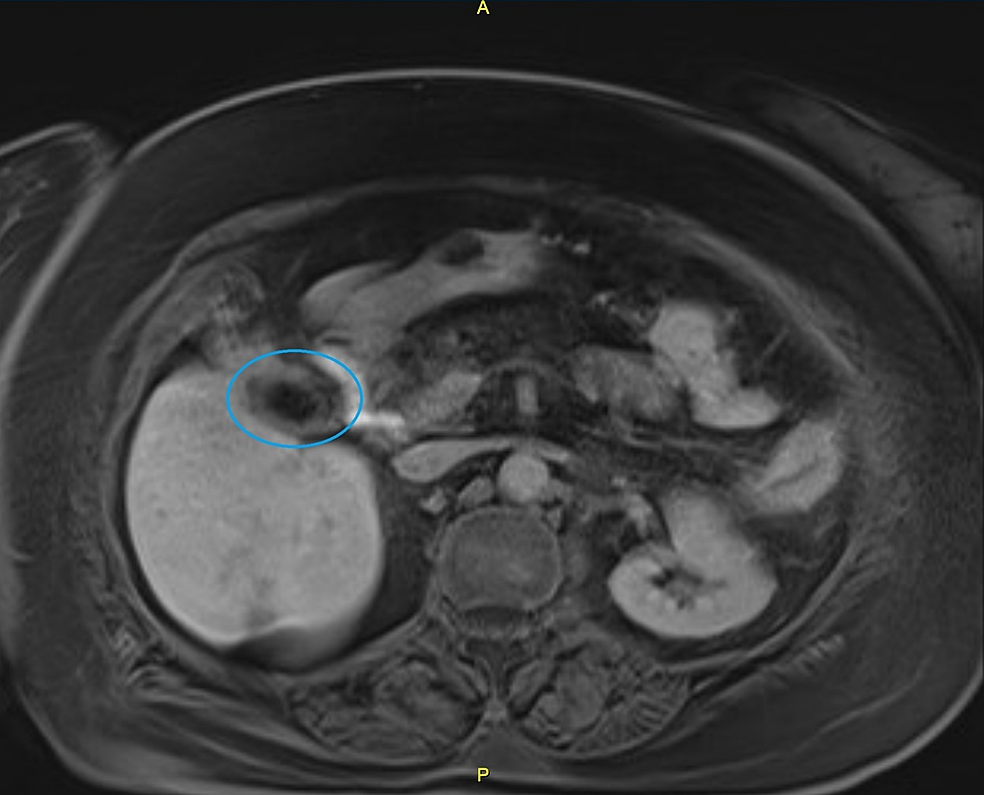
MRI abdomen: the encircled area highlights the gallbladder, which has an asymmetrically thickened wall with a linear, crescent-like signal void in the medial aspect
